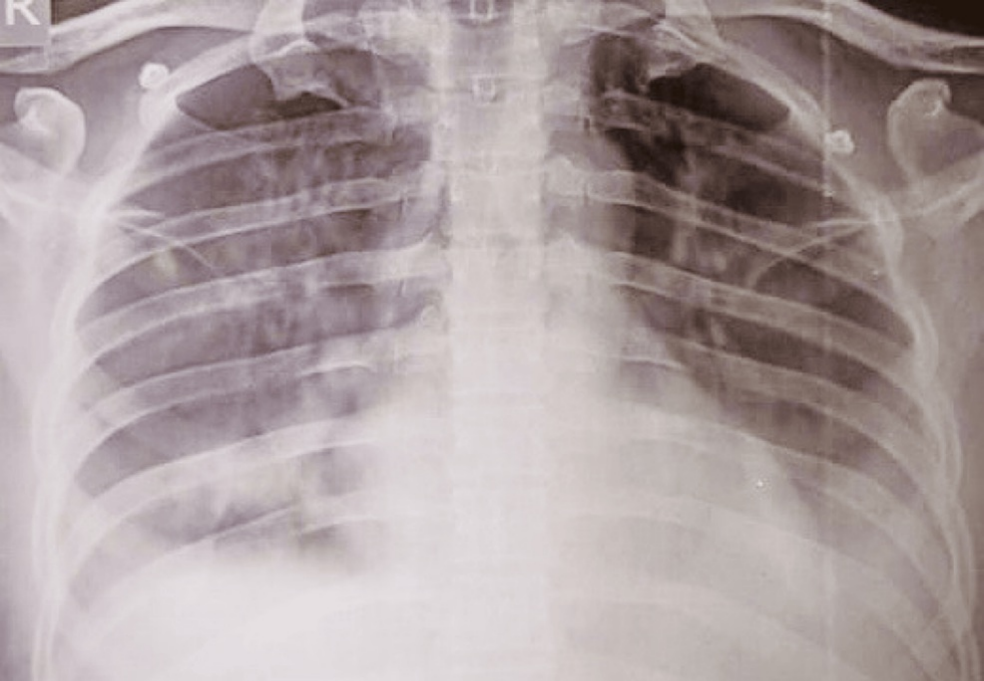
Chest X-ray of the patient demonstrating bilateral heterogenous infiltrates suggestive of acute respiratory distress syndrome (ARDS)
